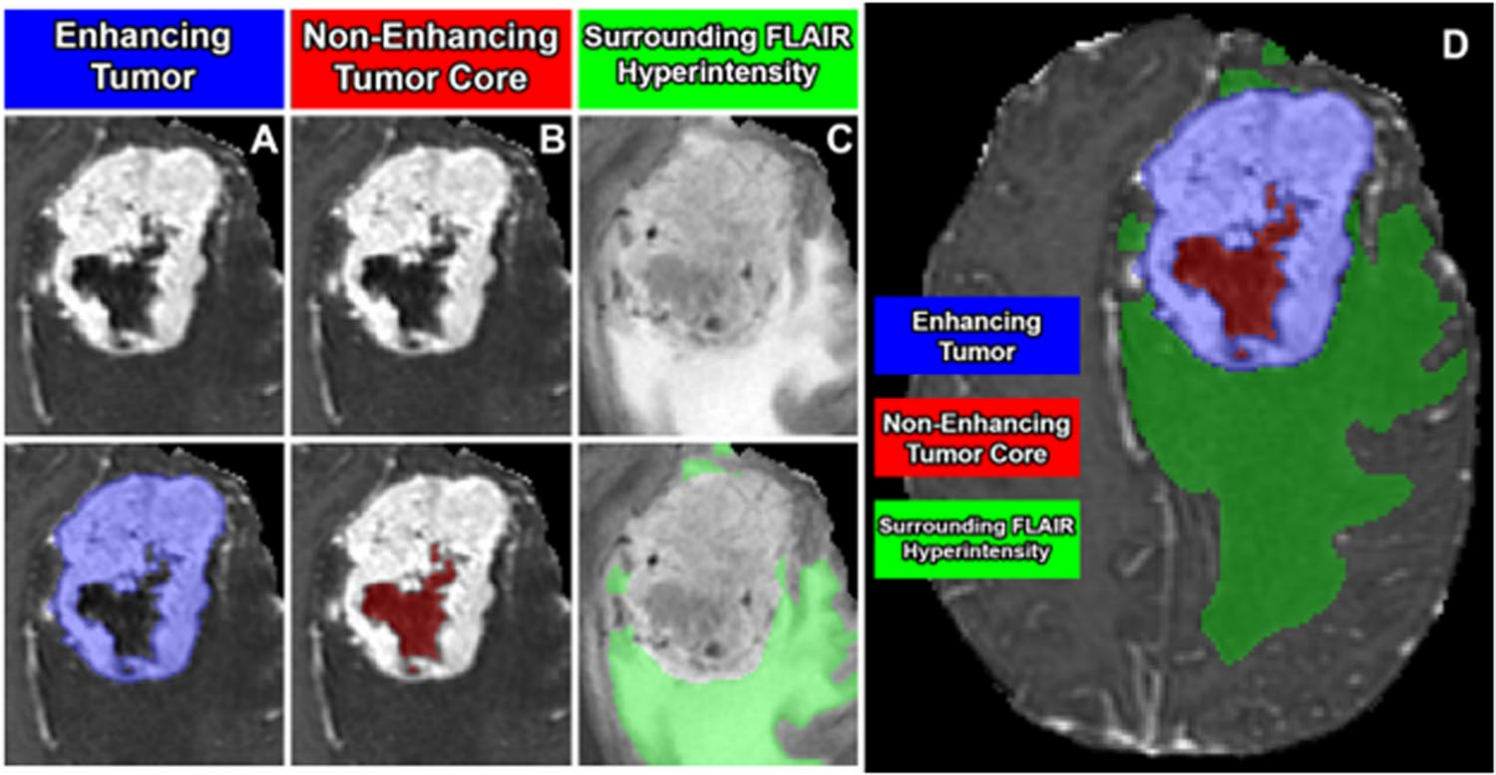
Meningioma sub-compartments considered in the BraTS Pre-operative Meningioma Dataset. Image panels A-C denote the different tumor sub-compartments included in manual annotations; (A) enhancing tumor (blue) visible on a T1-weighted post-contrast image; (B) the non-enhancing tumor core (red) visible on a T1-weighted post-contrast image; (C) the surrounding FLAIR hyperintensity (green) visible on a T2/FLAIR-weighted image; (D) combined segmentations generating the final tumor sub-compartment labels provided in the BraTS Pre-operative Meningioma Dataset.
